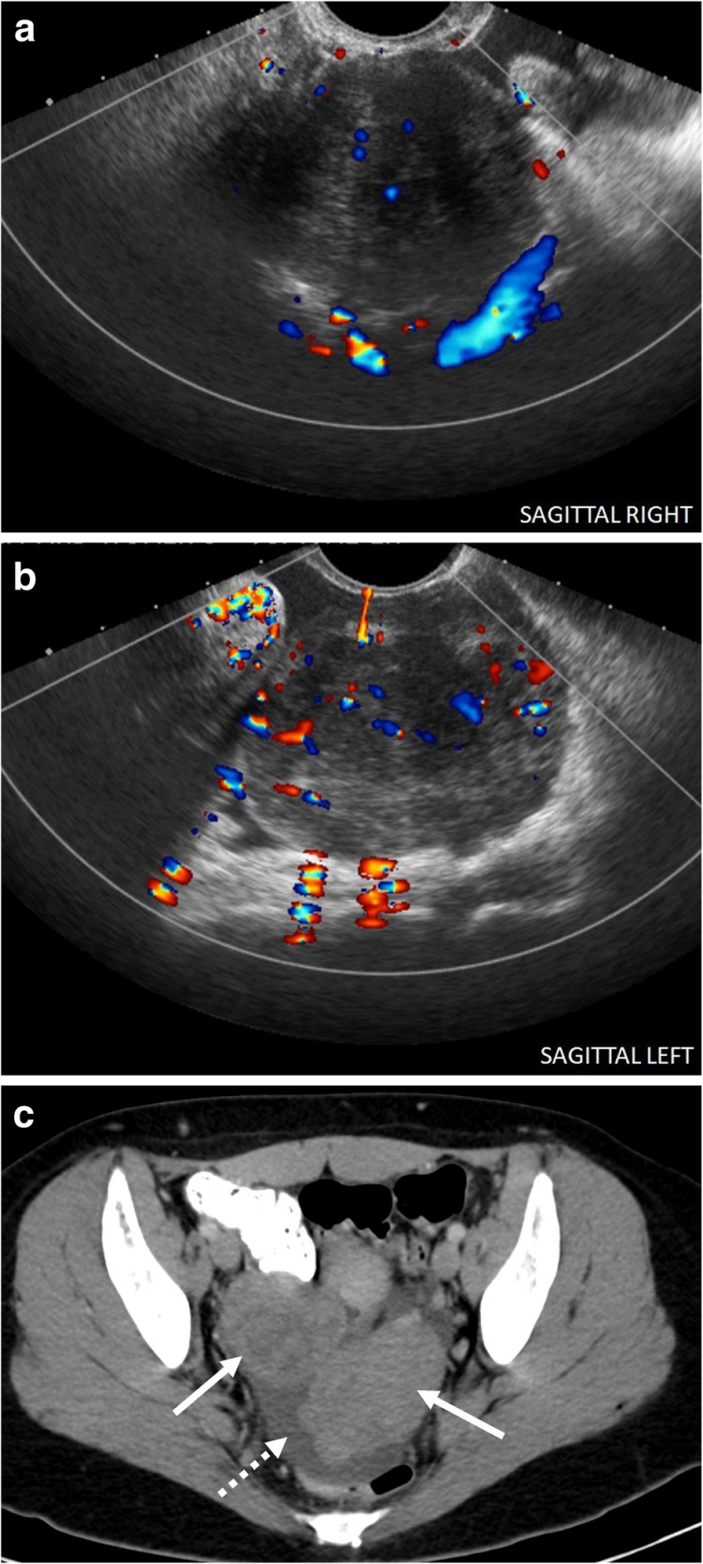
Bilateral ovarian masses. A 23-year-old female presenting with pelvic pain. Sagittal color Doppler images through the (a) right and (b) left ovaries demonstrate heterogeneous enlargement of the bilateral ovaries containing internal flow, greater on the left. c Subsequent contrast-enhanced CT shows markedly enlarged bilateral ovaries (solid arrows) with adjacent pelvic free fluid (dashed arrow). Subsequent exploratory laparotomy with bilateral salpingo-oophorectomy revealed Burkitt lymphoma
